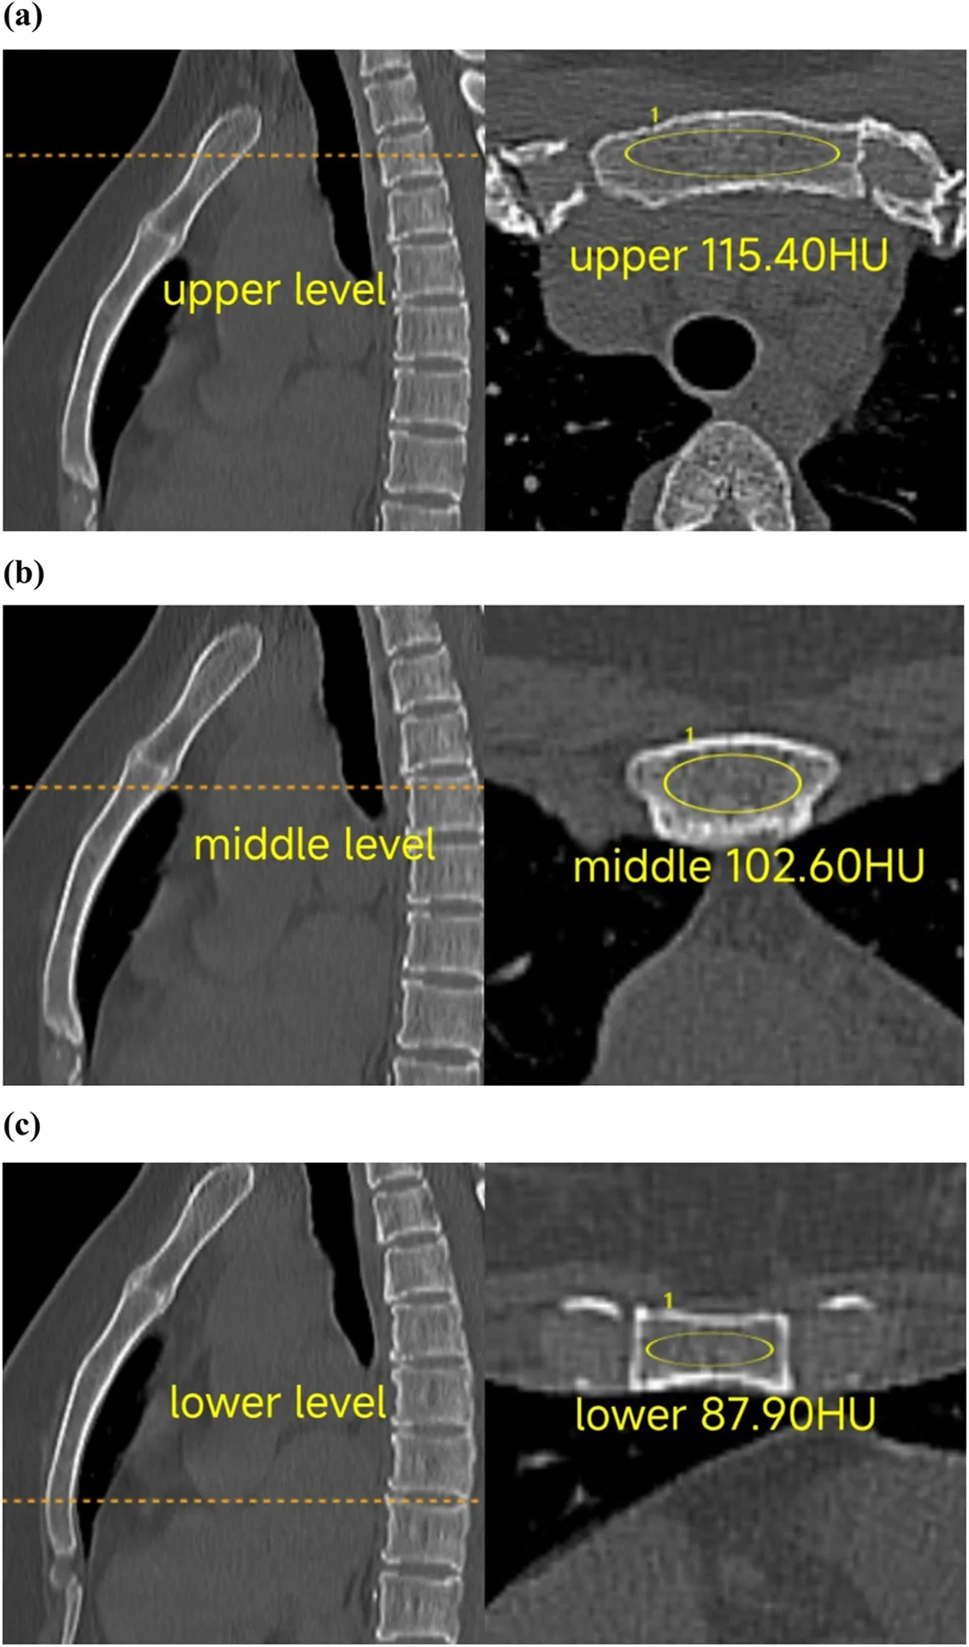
Methods for measuring CT values at different levels on chest CT scans. a CT scan of upper level of the sternum in the sagittal plane. b CT scan of middle level of the sternum in the sagittal plane. c CT scan of lower level of the sternum in the sagittal plane
